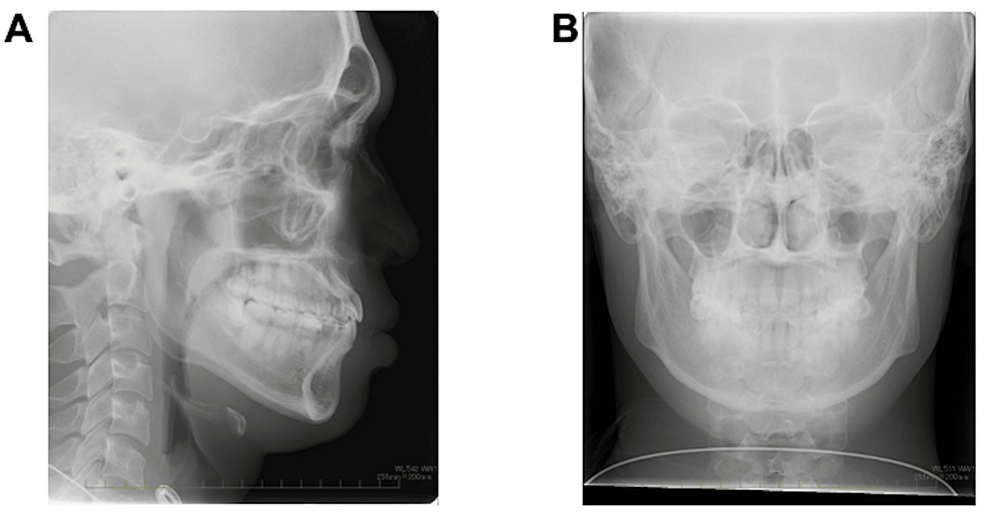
Cephalometric radiographs obtained after orthodontic treatment A: lateral cephalometric radiography performed obtained after orthodontic treatment; B: frontal cephalometric radiography performed after orthodontic treatment
